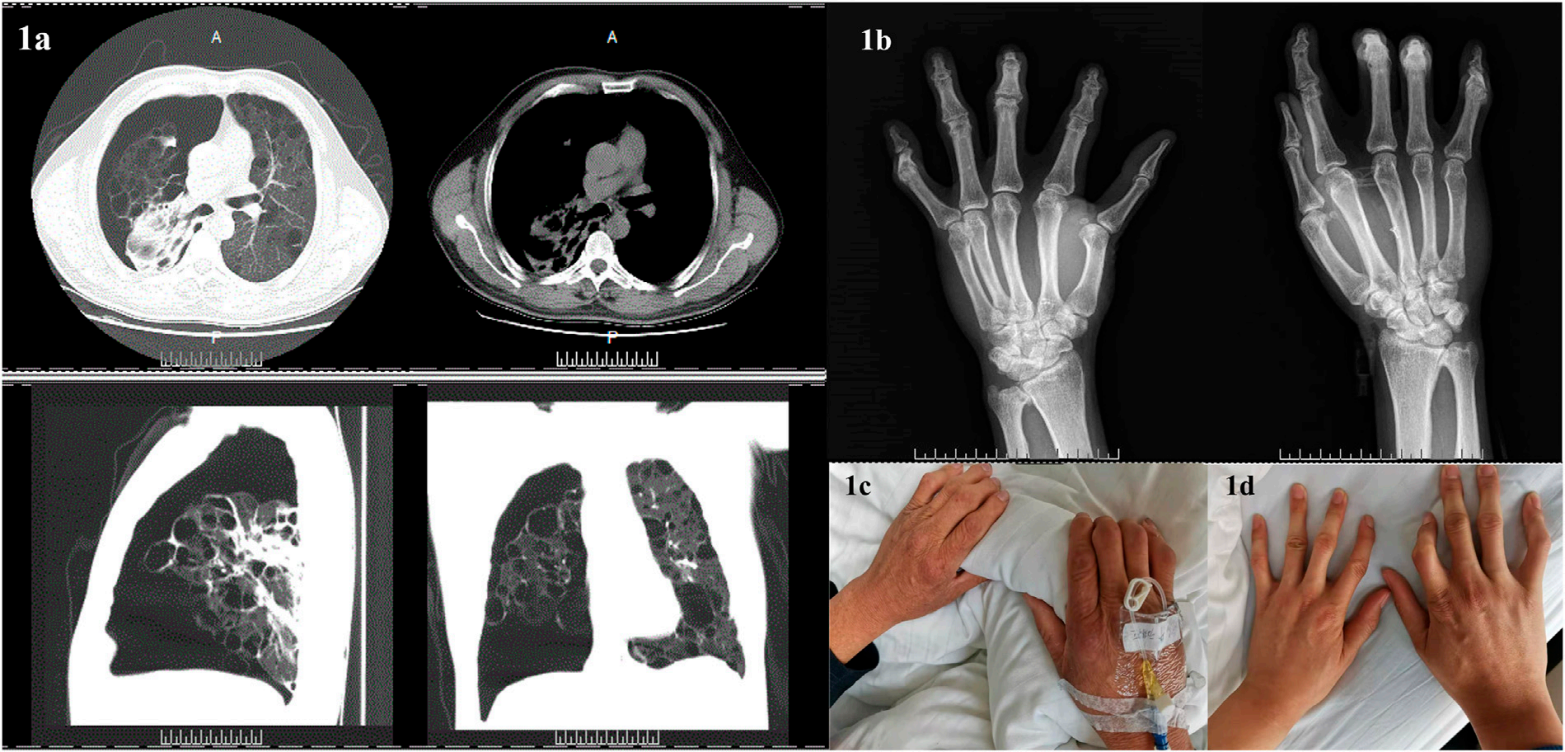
Clinical characteristics of the patient and family members. (A): Computed tomography of the patient’s chest indicated spontaneous pneumothorax on the right side and cyst and bullae in both lungs. (B): Digital radiography in both patient’s hands revealed changes in both hands and wrists, suggesting degenerative changes or chronic inflammatory lesions. (C): The patient’s hands. (D): The hands of the patient’s son.
